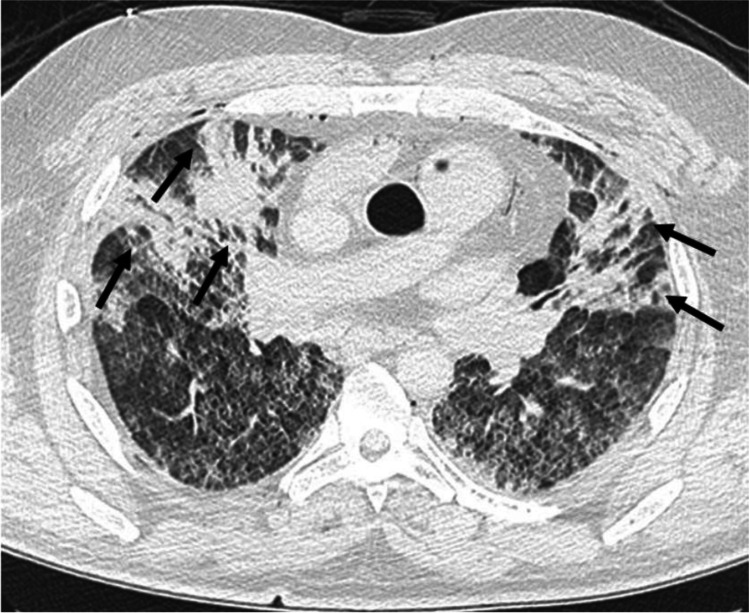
Post-mortem computed tomography (PMCT) showing diffuse bilateral interstitial thickening and panlobar air space consolidations in anterior segments of each lobes
